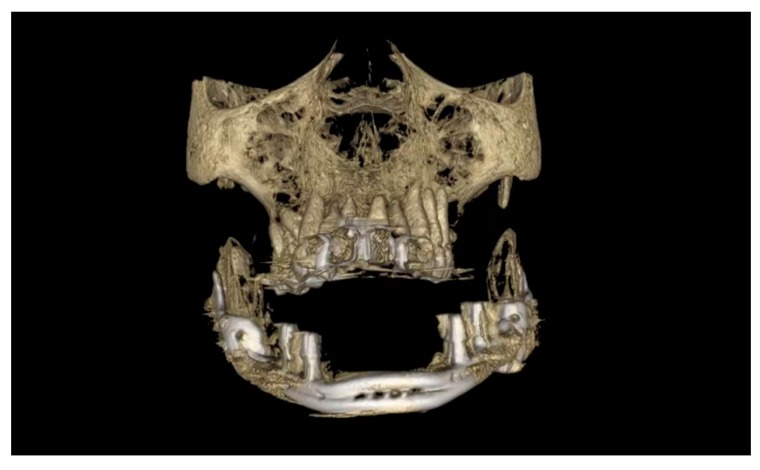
Computerized tomography (CT) after 2 years.
